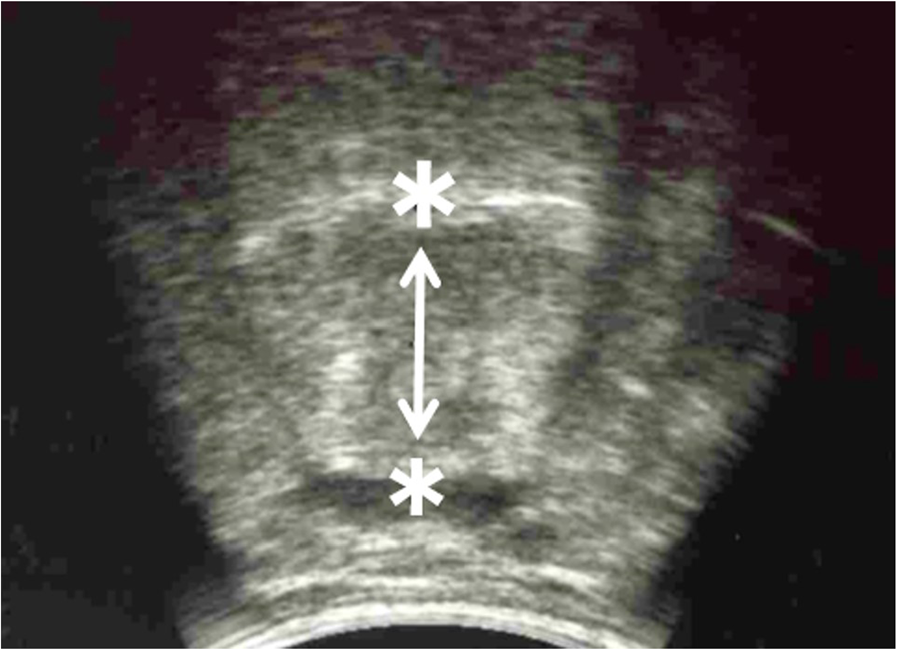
Tongue thickness measured by ultrasonography. Tongue thickness was defined as the distance from the upper boundary of the mylohyoid raphe to the upper surface of the tongue, including the geniophyoid and genioglossus muscles
